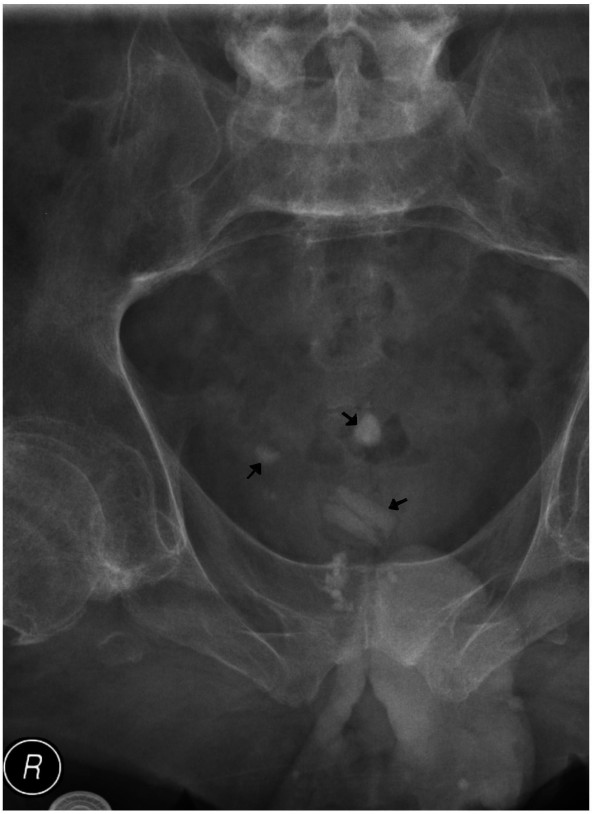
X-ray of urinary bladder taken on 09 June 2008 reveals one large and two small calculi (arrow).
